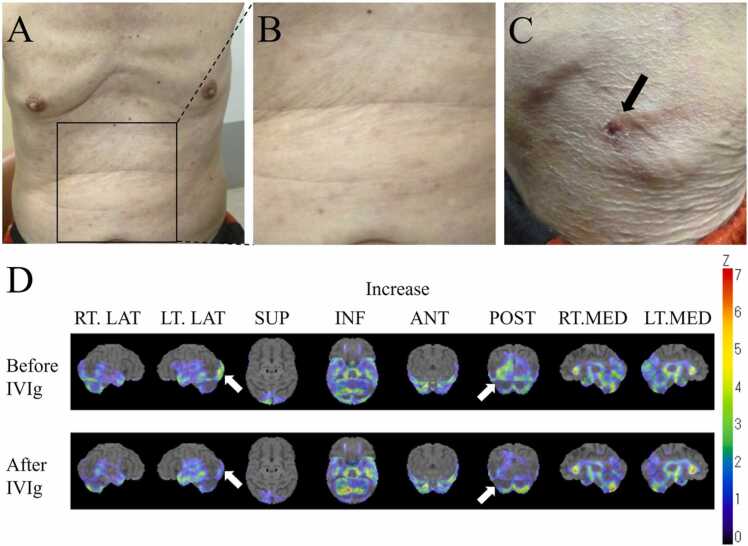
Images of the patient. The patient developed maculopapular rash on the trunk (A, B), back, hip and extremities. Eschar was detected on the left knee (black arrow, C). ^99m^TcHM-PAO single photon computed emission tomography (PAO-SPECT) was performed on the 5th hospital day before initiating intravenous immunoglobulin (IVIg). We detected increased cerebral blood flow in left occipital lobe, using three-dimensional stereotactic surface projection (3D-SSP) analysis (white arrows, D above). On PAO-SPECT performed on the 19th hospital day after the treatment with IVIg, the increased blood flow in left occipital lobe was reduced (white arrows, D below). The both PAO-SPECTs were performed using the following protocol: 1110 MBq of ^99 m^Tc-HMPAO was administered, and imaging was performed on a Dual-head SPECT scanner: Symbia T2 (Siemens Japan) starting about 5 min later. Color coding represents the statistical significance (Z-score) of the increase in regional cerebral blood flow compared with age-matched normal controls. Abbreviations; RT, right; LT, left; LAT, lateral; SUP, superior; INF, inferior; ANT, anterior; POST, posterior; MED, medial.
